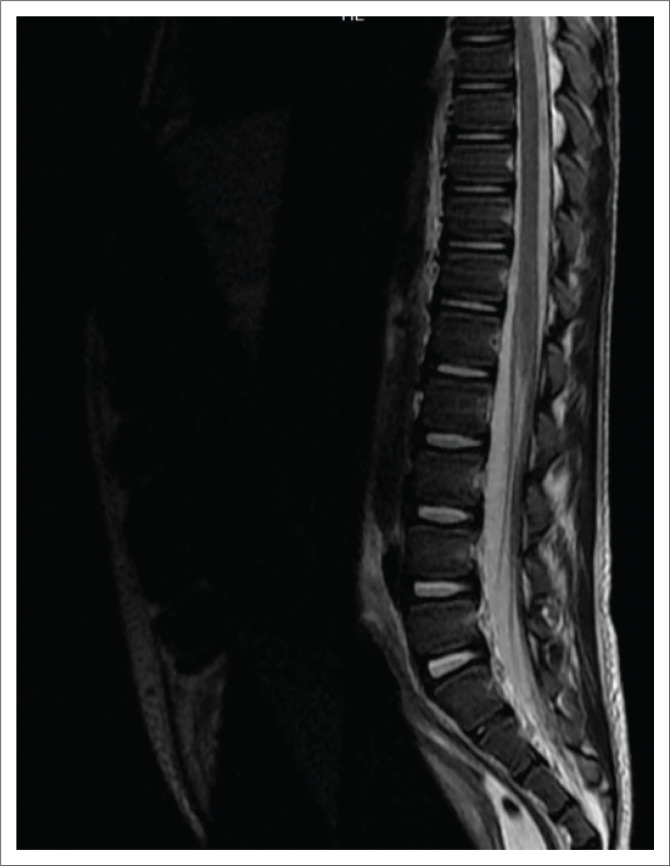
Sagittal T2-weighted magnetic resonance imaging sequence of the lower thoracic and lumbar spine demonstrating normal signal intensity in the spinal cord and conus medullaris.
